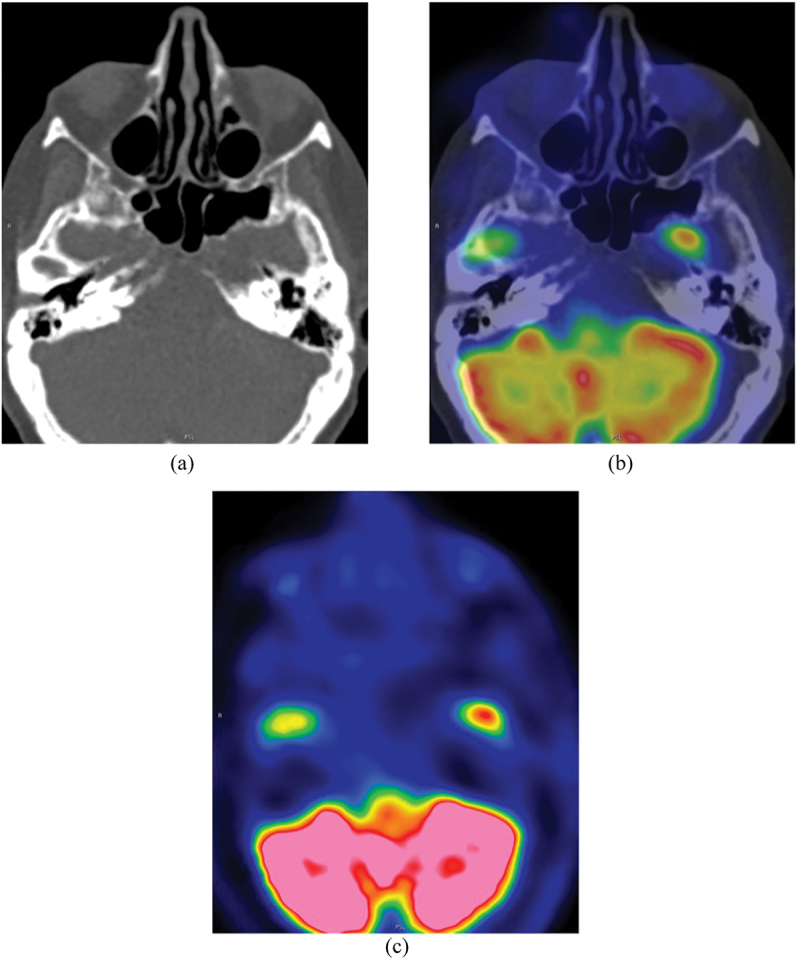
CT (a) and FDG-PET/CT (b, c) 4 months after methotrexate cessation showed continued disease regression.
